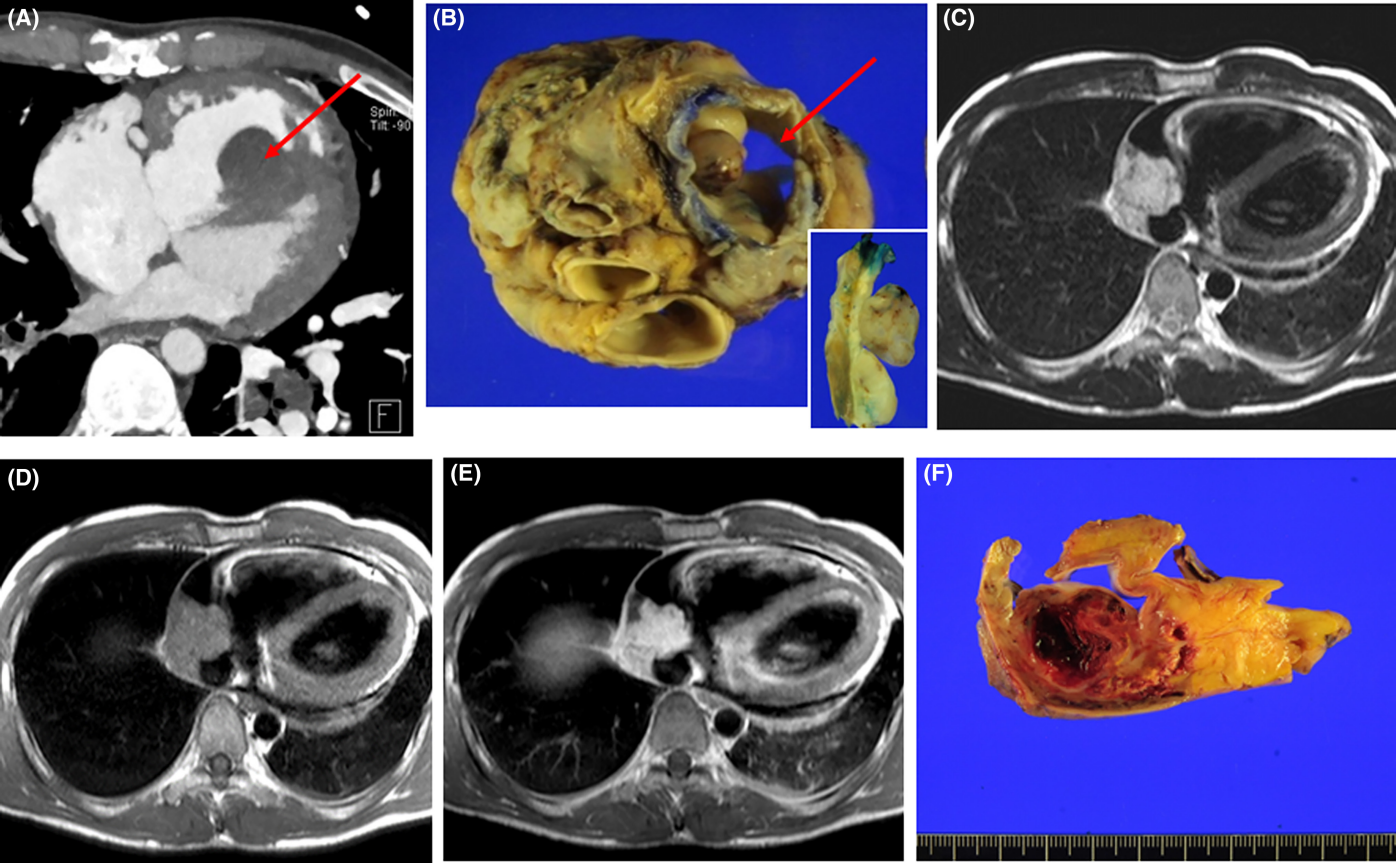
Radiologic and gross findings for the intimal sarcoma (A–B) and angiosarcoma (C–F) patients treated with a heart transplantation. (A, B). A 17 years‐old man received a heart transplantation due to a left atrial mass. A cardiac CT (A) showed a non‐enhanced mass (red arrow) with a suspicion of a focal myocardial invasion. The patient underwent a heart transplantation, and the polypoid mass was found to have been present in the endocardium of the left atrium (B, red arrow). Grossly, the cut surface of the tumor was gray tan and fibrotic without hemorrhage (inset). (C–F). A 66 years‐old woman underwent a heart transplantation due to a right atrial mass. Magnetic resonance images revealed that the mass (white arrow) was T2 high signal intensity (C), T1 low signal intensity (D) and T1 enhanced signal intensity (E), suggestive of an angiosarcoma. (F) Grossly, the mass filled the entire right atrium (RA) and had a hemorrhagic cut surface.
